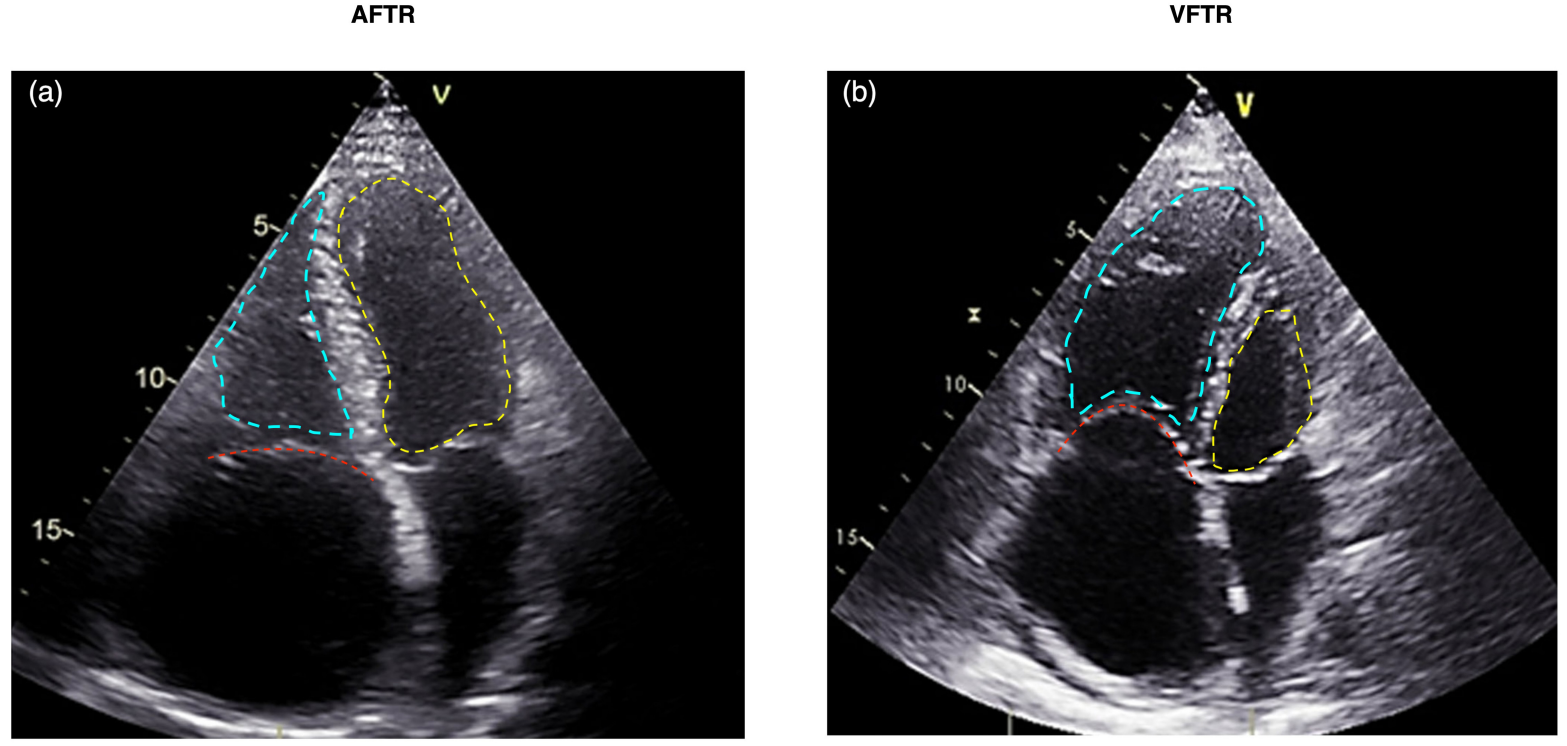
Echocardiographic comparison of AFTR (a) versus VFTR (b). The
